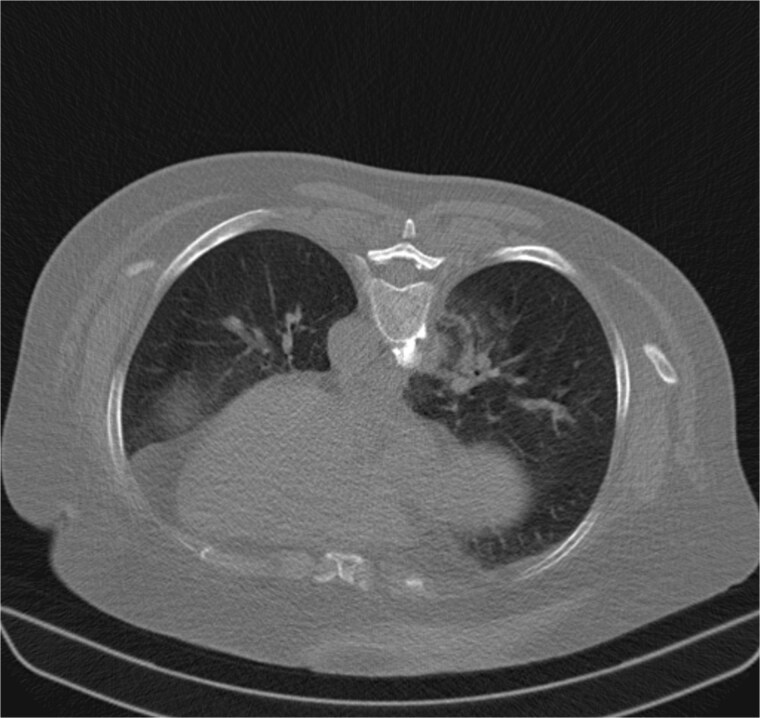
Axial non-contrast chest CT obtained immediately after ablation demonstrates expected parenchymal changes. The ablation zone shows central alteration, with a circumferential rim of ground-glass opacity outlining the thermal margin. No pneumothorax or hemorrhage was observed.
